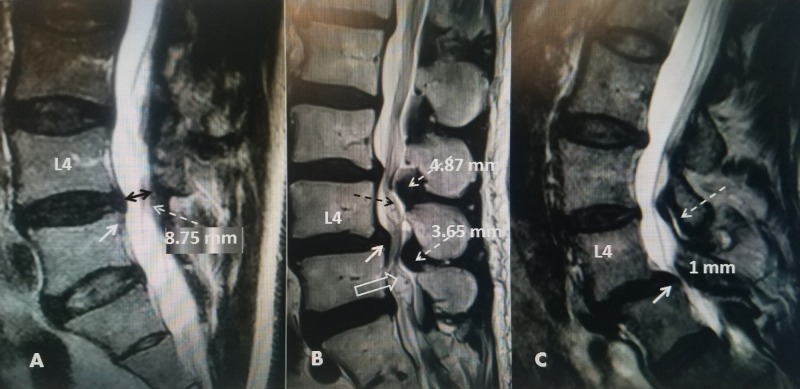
Sagittal T2 MRI scans compared in three different patients with different grades of spinal stenosis. A: Sagittal T2 MRI showing mild stenosis at L4-5 (double-headed black arrow) primarily secondary to ligamentous narrowing of the L4-5 canal posteriorly (dashed white arrow). There is minimal evidence of spondylolisthesis (solid white arrow) but the sagittal canal diameter at L4-5 is reduced to 8.75 mm (dashed white arrow). B: Sagittal midline T2 MRI scan with the MRI plane made directly through the posterior spinous processes showing grade 1 spondylolisthesis at L4-5 (solid white arrow). There is marked posterior ligamentous hypertrophy and encroachment at both L 3-4 and L 4-5 (dashed white arrow). The mid-sagittal canal diameter is 4.87 mm at L3-4 and 3.65 mm at L4-5 (dashed white arrows) The swollen, matted nerve roots (open white arrow) seen just below the L4-5 stenosis are characteristic signs of nerve root edema and compression seen with severe canal stenosis. The swollen cauda equina roots (dashed black arrow) above the stenosis is also characteristic of high-grade stenosis. C: Sagittal T2 MRI showing more severe grade 1-2 spondylolisthesis (solid white arrow) and marked lumbar spinal canal narrowing at L4-5. The sagittal dimension at L4-5 is 1 mm (solid white arrow). There is separate mild L3-4 posterior compression (dashed white arrow).
